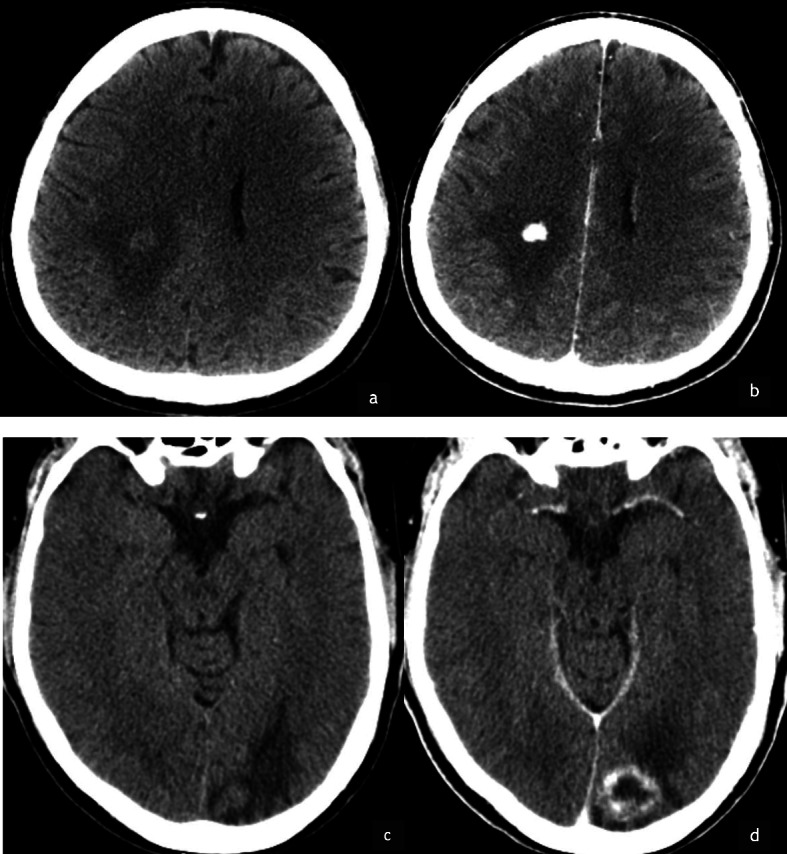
Pre and post contrast CT of the brain in a 33 year old female (a & b). White matter low attenuation is appreciated in the right parietal lobe in keeping with vasogenic oedema . Following contrast there is markedly avid enhancement (b) confirming intraparenchymal metastasis. Pre and post contrast axial CT of a 31 year old male (c & d) patient also demonstrates a similar morphology with avid peripheral enhancement of a left occipital lobe metastasis (d)
